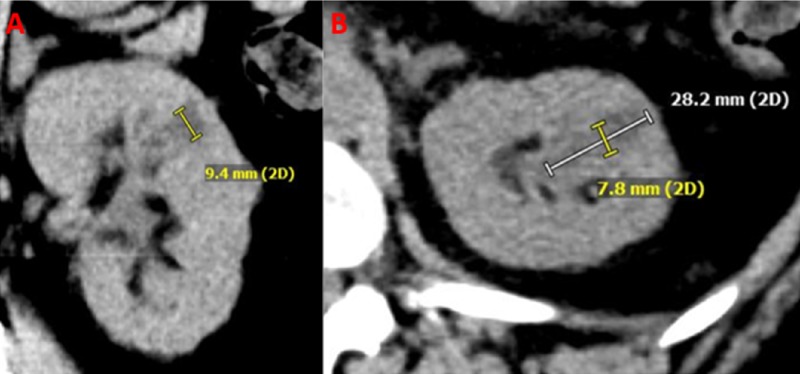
Tumor reduction in the post-embolization period. Coronal (A) and axial (B) images from a follow-up computed tomography (CT) three months post-embolization, shows approximately 90% reduction in the volume of the renal lesion.
